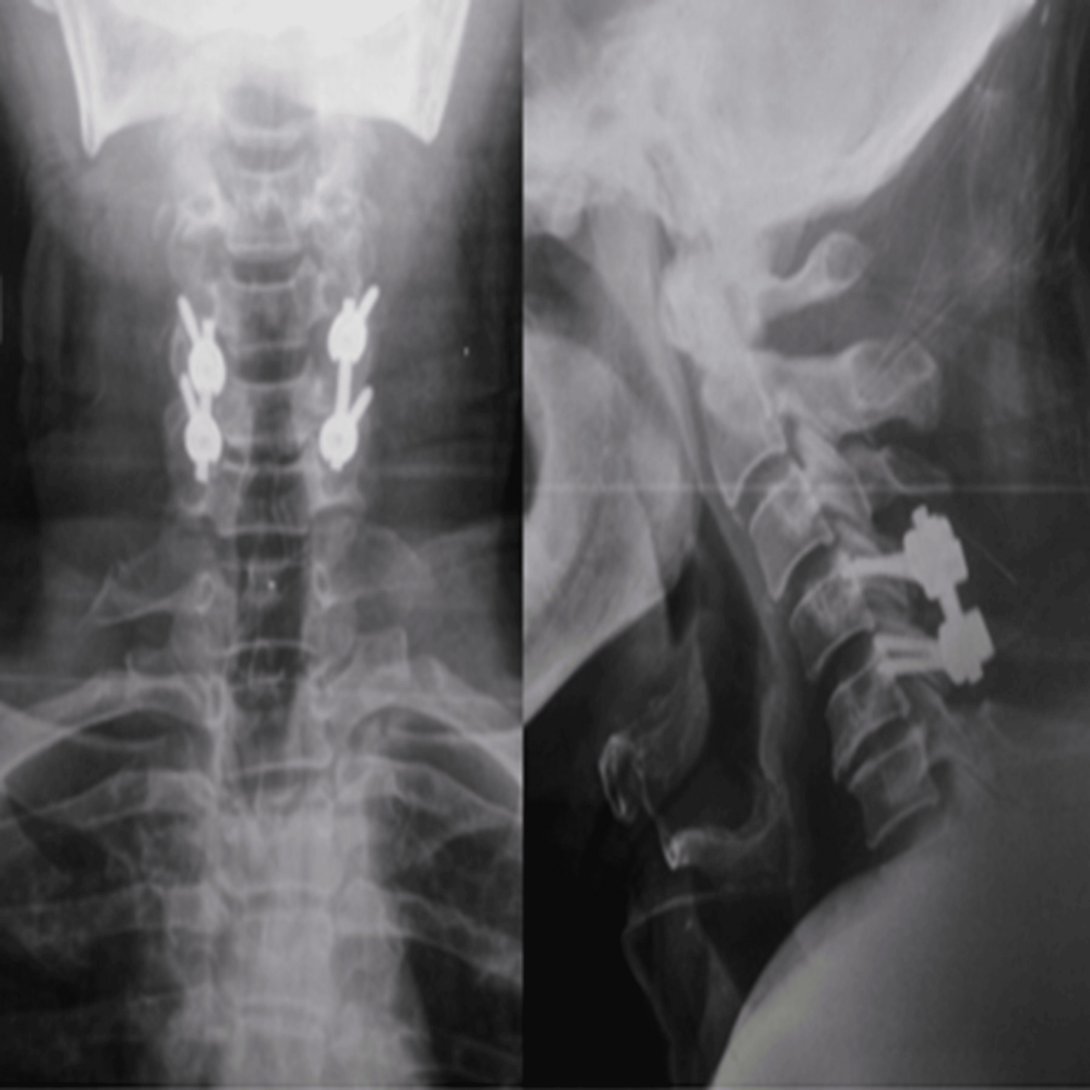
Postoperative plain X-ray of the cervical spine (A) AP view and (B) lateral view showing C4-C5 decompression and lateral mass fixation.
